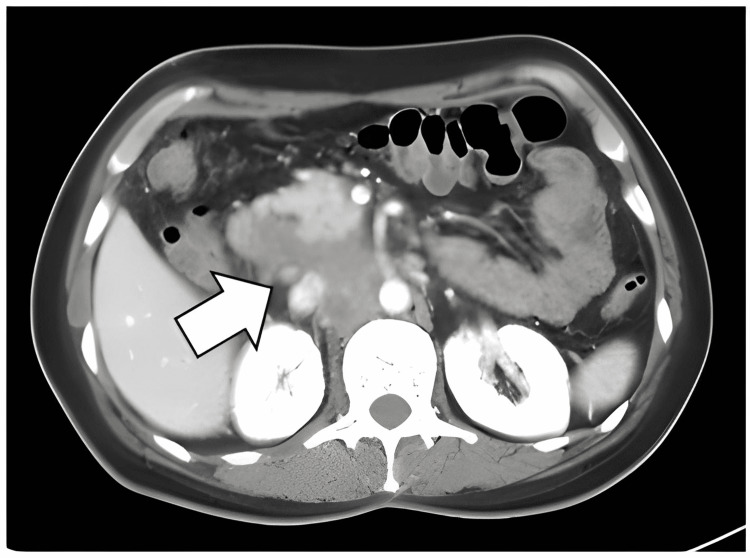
Abdominal CT-scan showed an alteration of pancreatic density with increased cephalic segment volume that included hypodense areas of necrotic aspect that contacted the duodenal wall.
